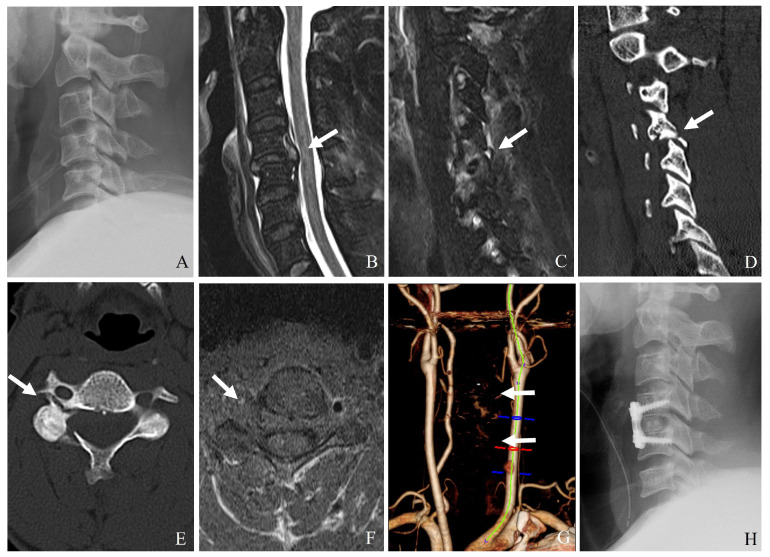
Plain X-ray (A), magnetic resonance imaging (B,C), and computed tomography (D,E) indicate unilateral facet subluxation, traumatic disc herniation, and fractures of the right facet, pedicle, and lamina at C4–5 (white arrows). Axial magnetic resonance imaging (F) and neck computed tomography angiography (G) reveal left vertebral artery injury (white arrows). The patient underwent combined anterior cervical discectomy, fusion, and posterior fixation of C4–5 (H).
